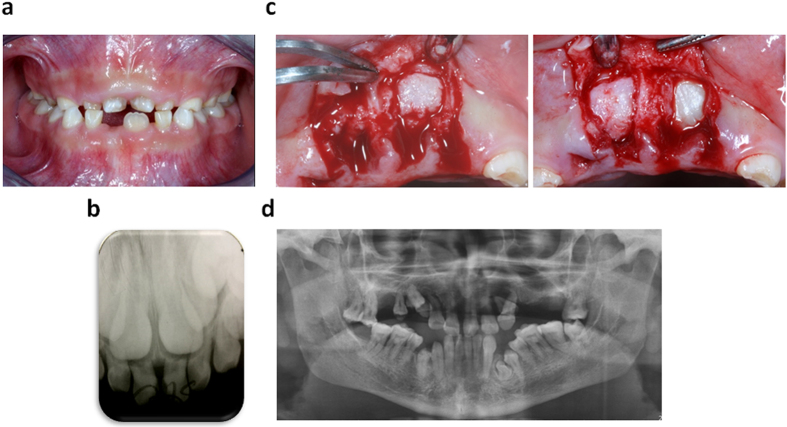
Retained deciduous teeth and multiple impacted permanent teeth in cleidocranial displasia patient. (a) Intraoral photograph pre-treatment. (b) Periapical film of retained primary maxillary incisors and their successors. (c) Unerupted maxillary central incisors covered by dental follicle were surgically exposed. (d) Panoramic radiograph of the patient’s father.
